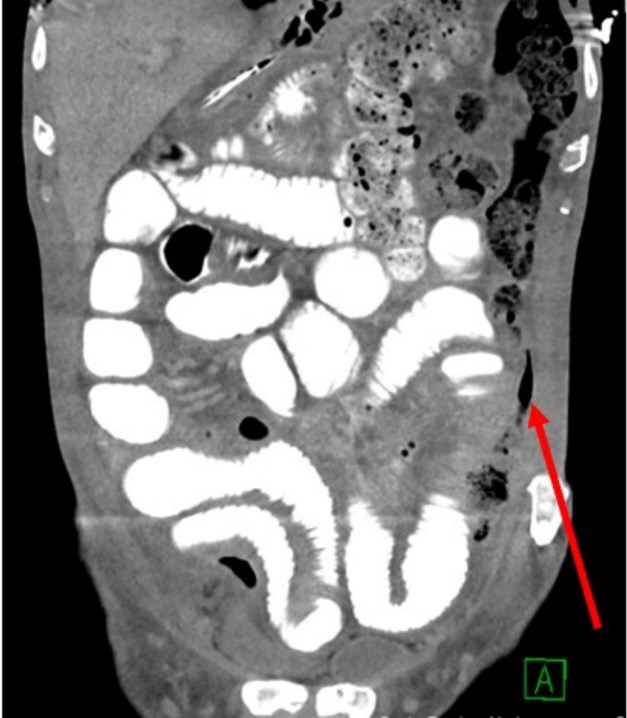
ASAP CT scan of abdomen after stabilization of electrolytes on suspicion of intestinal perforation.
